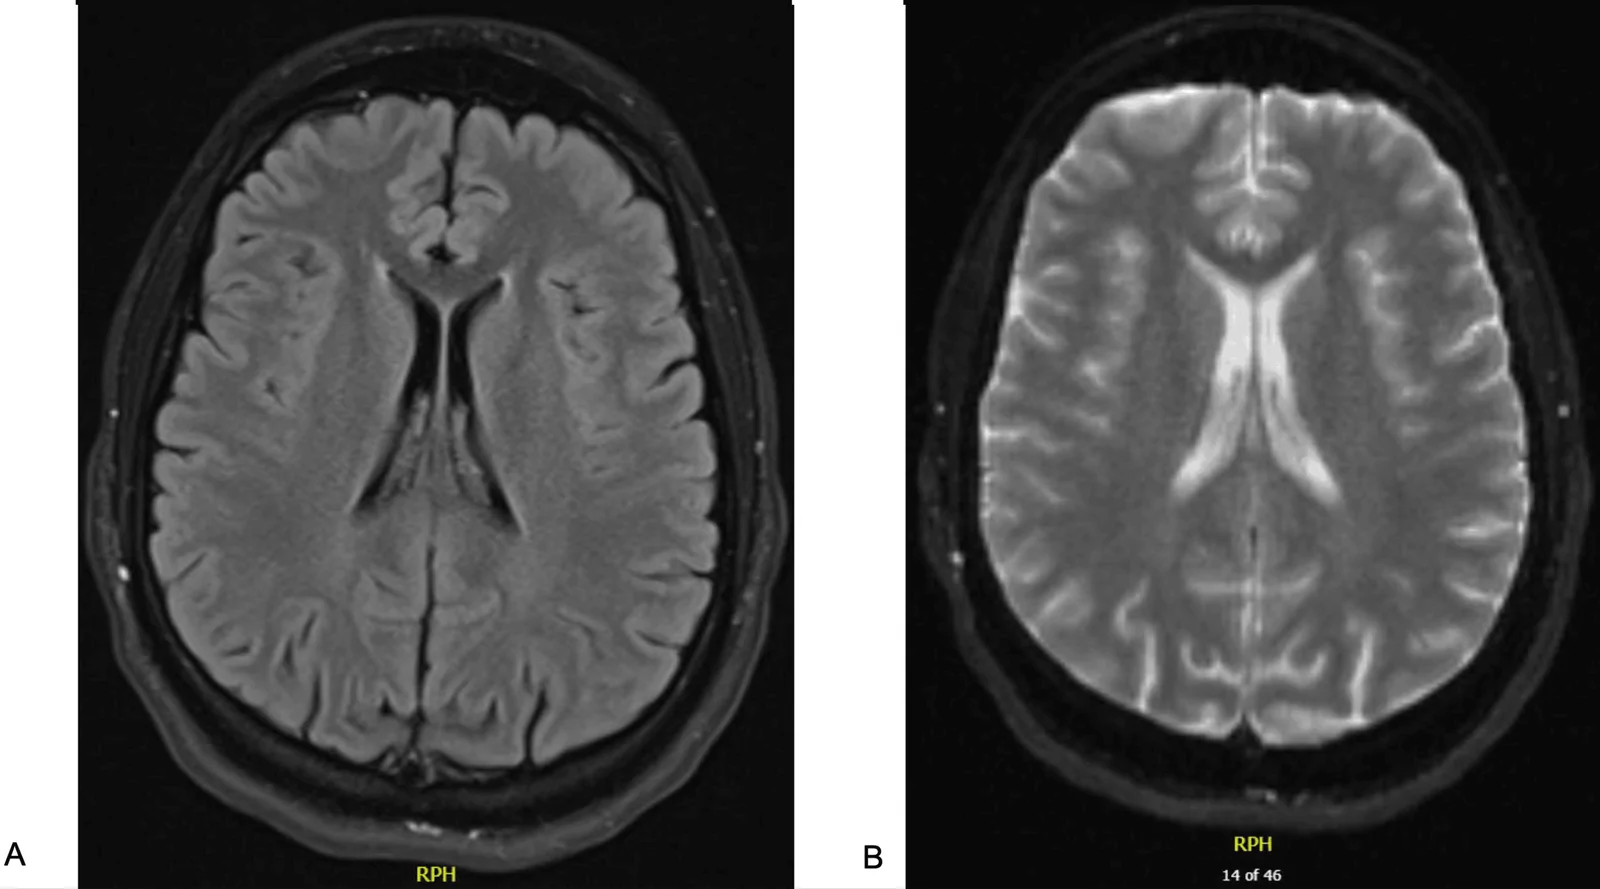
Representative brain magnetic resonance imaging during neurologic reassessment (A) Axial fluid-attenuated inversion recovery (FLAIR) image demonstrating no acute intracranial abnormality. (B) Axial diffusion-weighted imaging (DWI) demonstrating no diffusion restriction. These representative images were obtained during evaluation of recurrent neuropsychiatric symptoms and demonstrated no acute intracranial abnormality or diffusion restriction.
